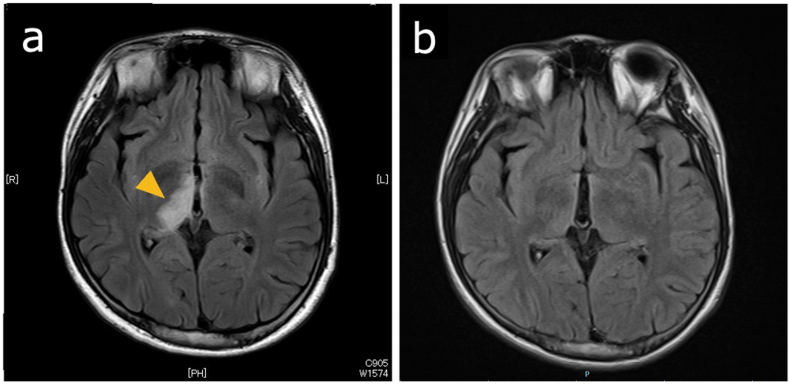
Magnetic resonance imaging (MRI) findings before and after diagnosis and treatment for myelin oligodendrocyte glycoprotein antibody-associated disease in a 14-year-old Japanese boy with acute macular neuroretinopathy associated with optic neuritis. Brain MRI reveals demyelinating lesions extending from the right thalamus to the midbrain (a). After treatment with two courses of intravenous methylprednisolone (1000 mg daily for 3 days), seven plasma exchange sessions, one intravenous immunoglobulin treatment session, and azathioprine, follow-up MRI shows reduced demyelinating lesions (b).
